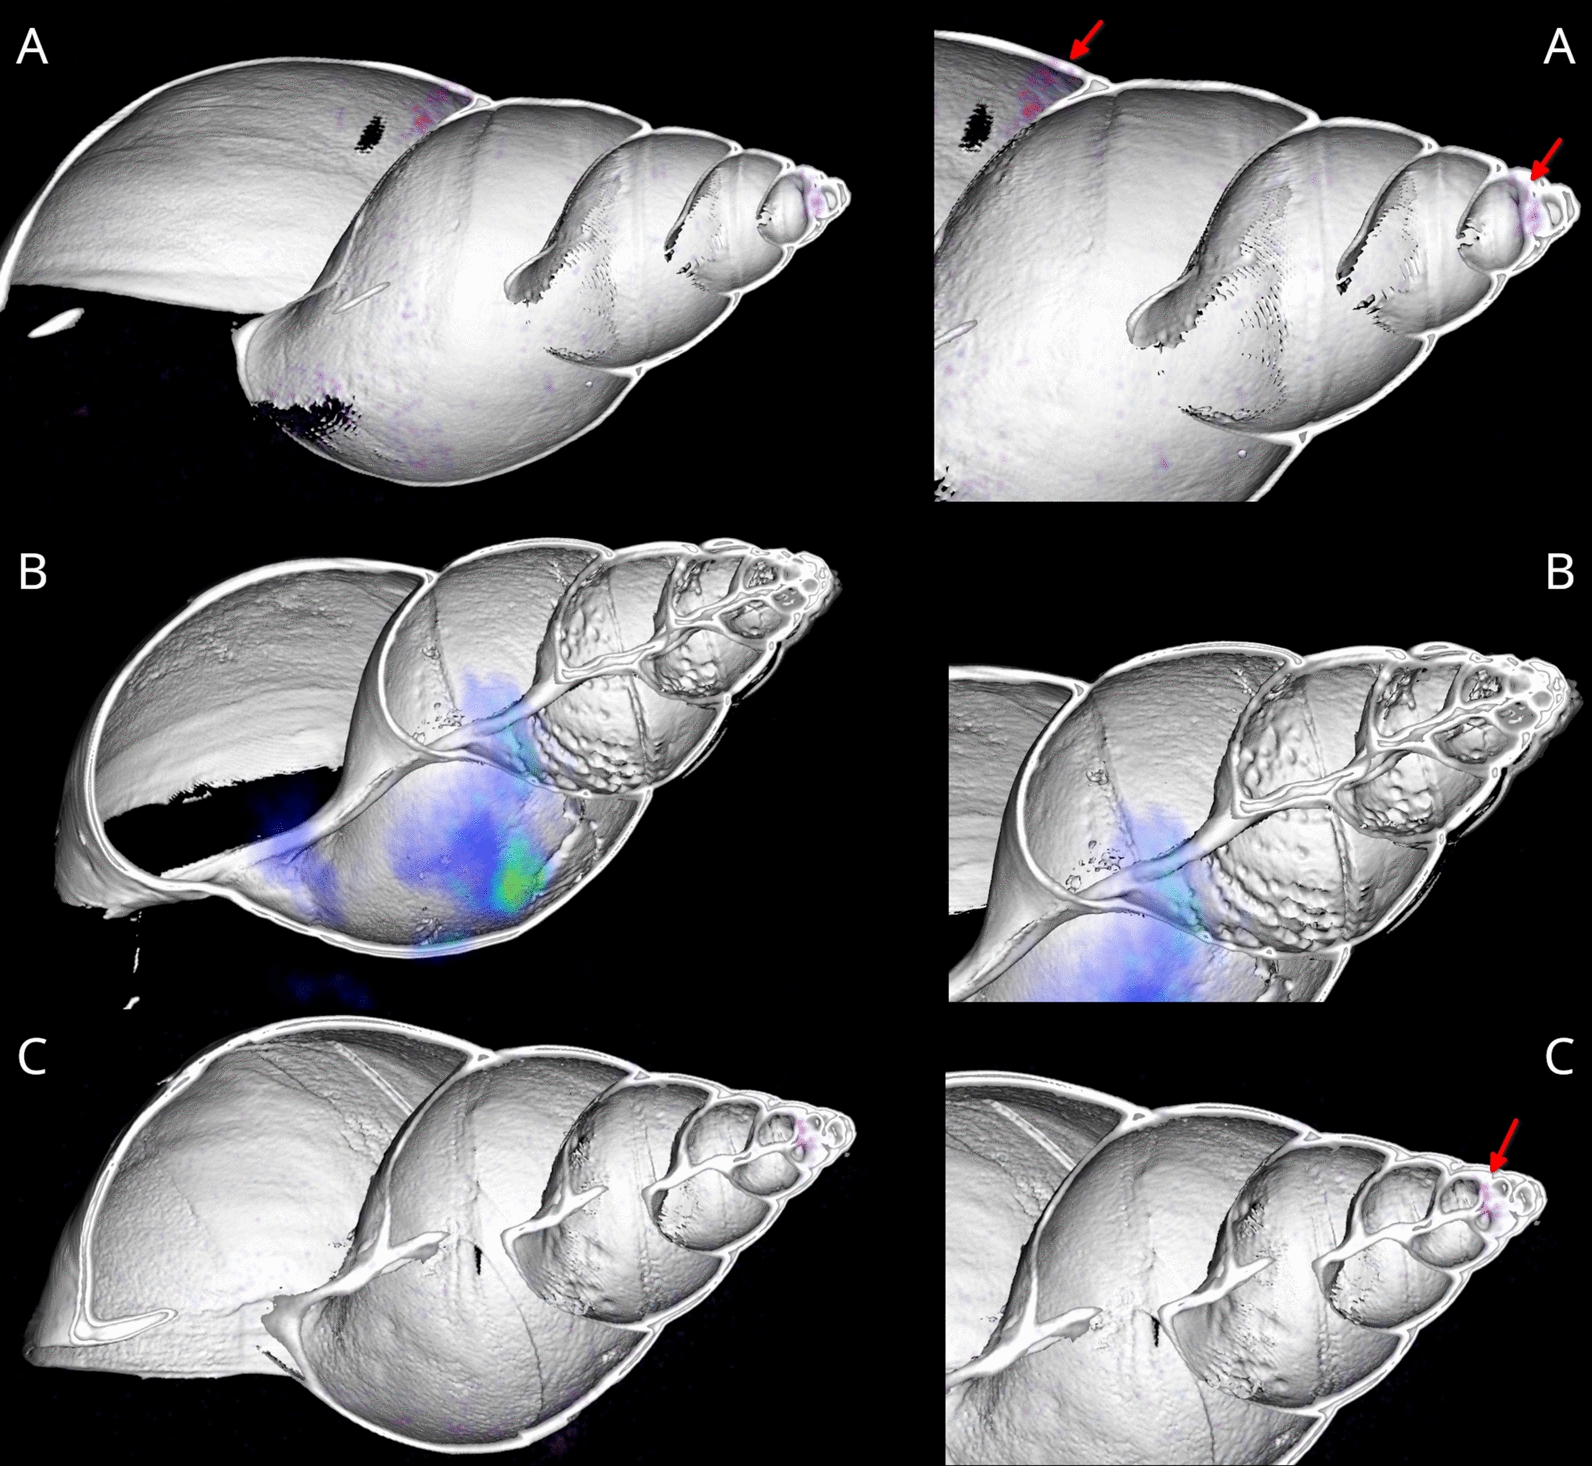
PET/CT image of two *Lissachatina fulica* snails (A, C) and one control (B) scanned 60 min after injecting ^18^F-FDG-radiolabeled *Angiostrongylus vasorum* larvae. A, top; red arrows: activity in the digestive tract and the digestive gland. B, middle: control. C, bottom: digestive gland visible
